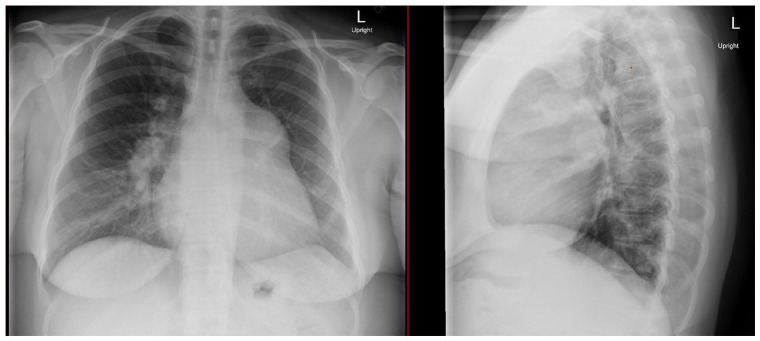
Chest X-ray demonstrating enlarged cardiac silhouette with mediastinal shift and large central pulmonary arteries.
